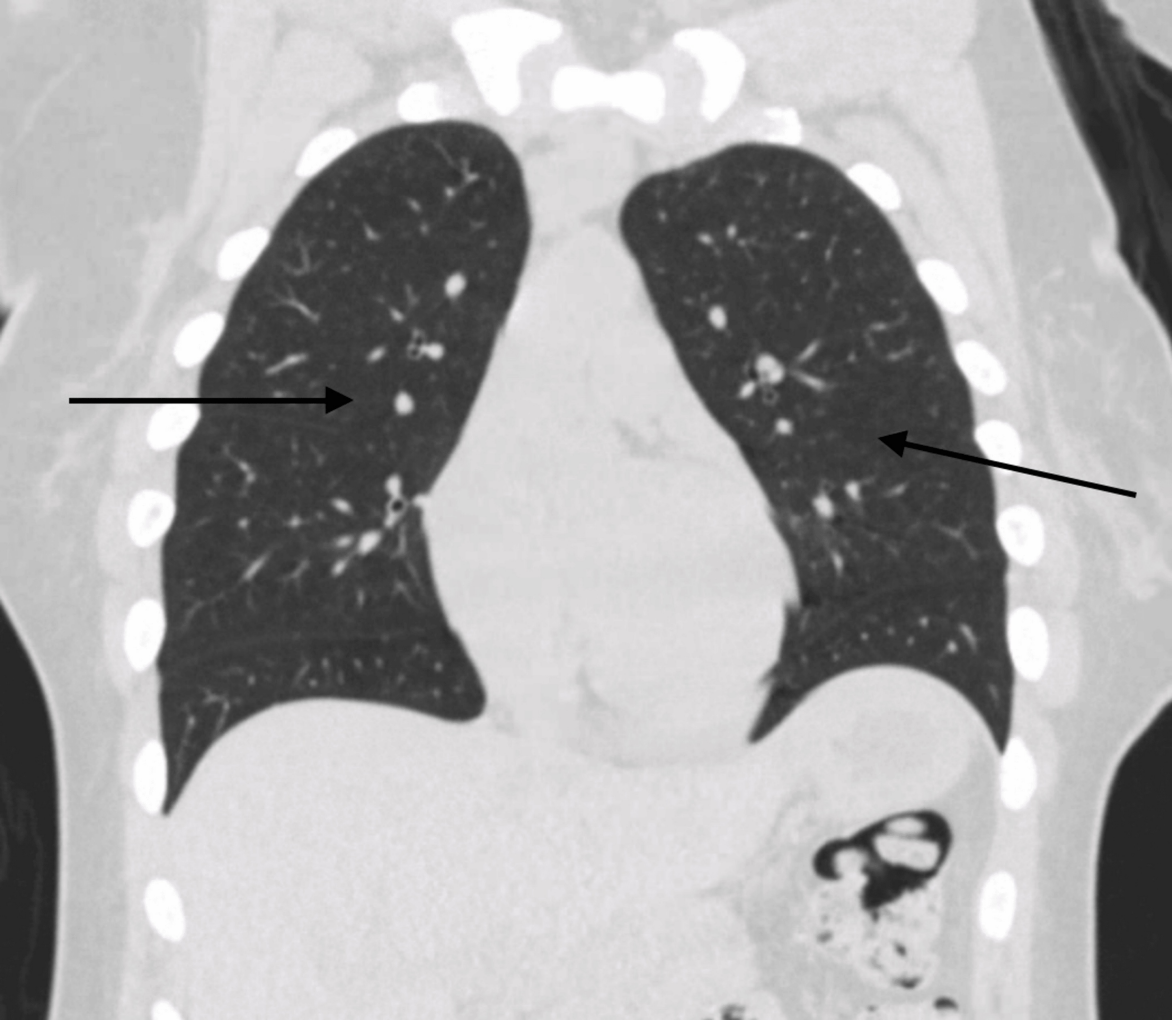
Repeat computed tomography of the chest two months later. Black arrows showing complete resolution of ground-glass opacities
